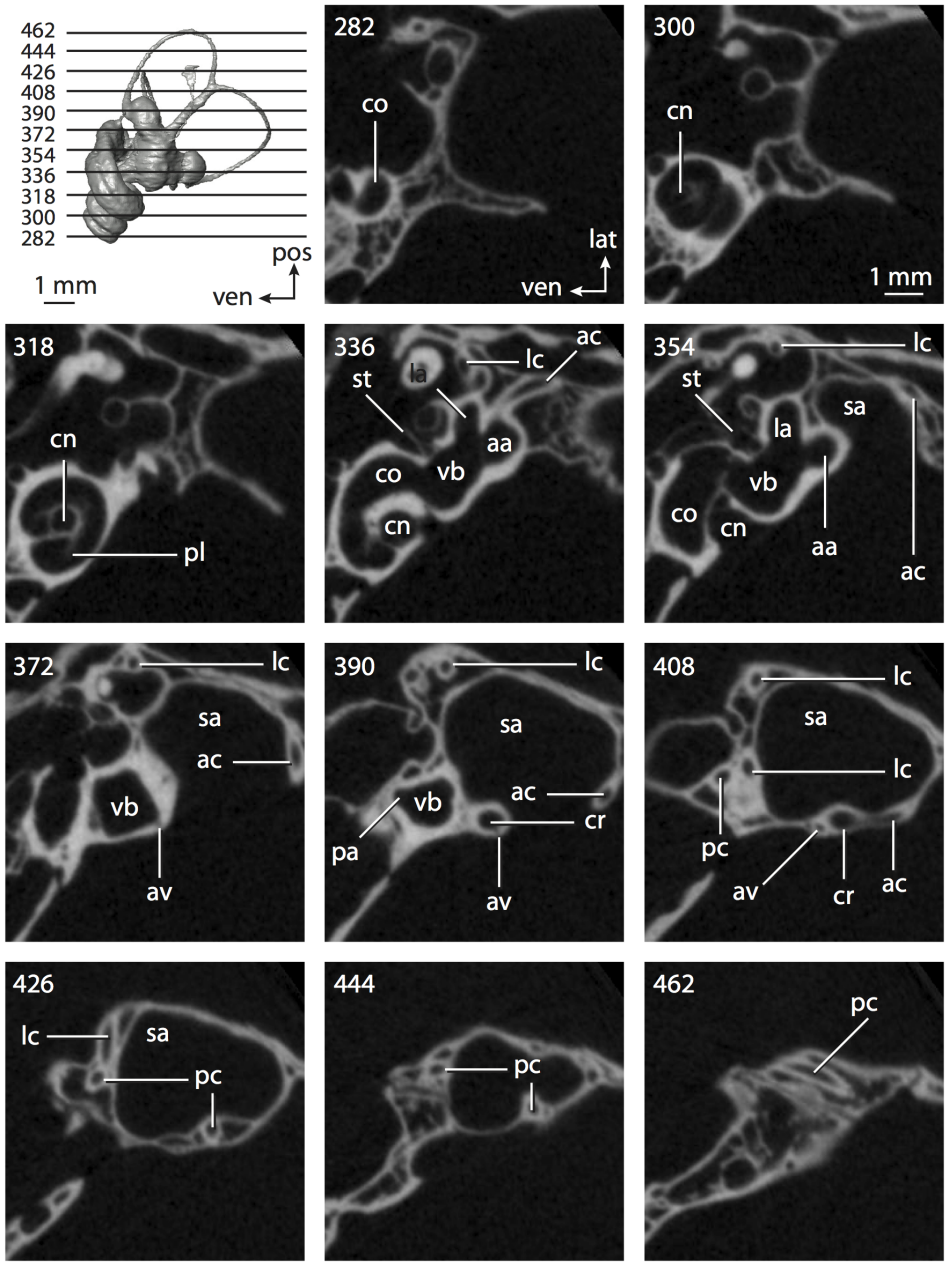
CT slices through ear region of*Tupaia glis.* Abbreviations listed at the end of the Materials and Methods section.
